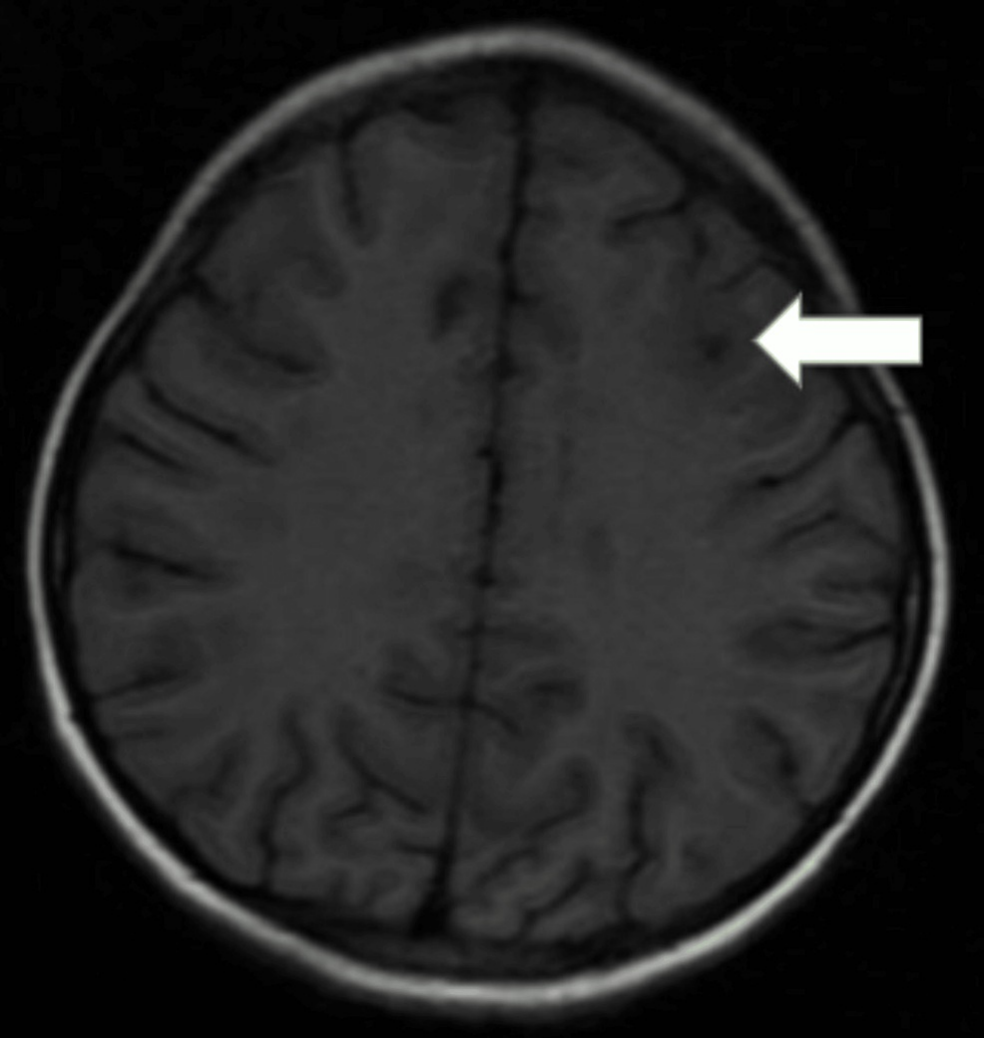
Axial T1-weighted MRI showing hypointensities in the subcortical white matter. MRI: magnetic resonance imaging
